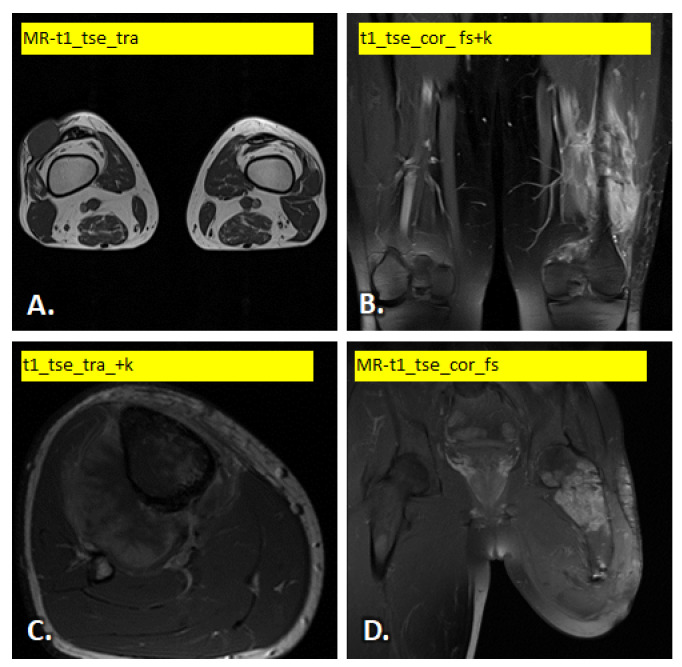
Example of T1-weighted MRI scan variants: (A) soft tissue tumor in the lateral joint capsule of the right knee, (B) bone tumor (Ewing) in the lower end of the left femur, (C) bone tumor on the proximal posterior side of the right tibia and (D) bone tumor in the trochanteric area of the left femur.
